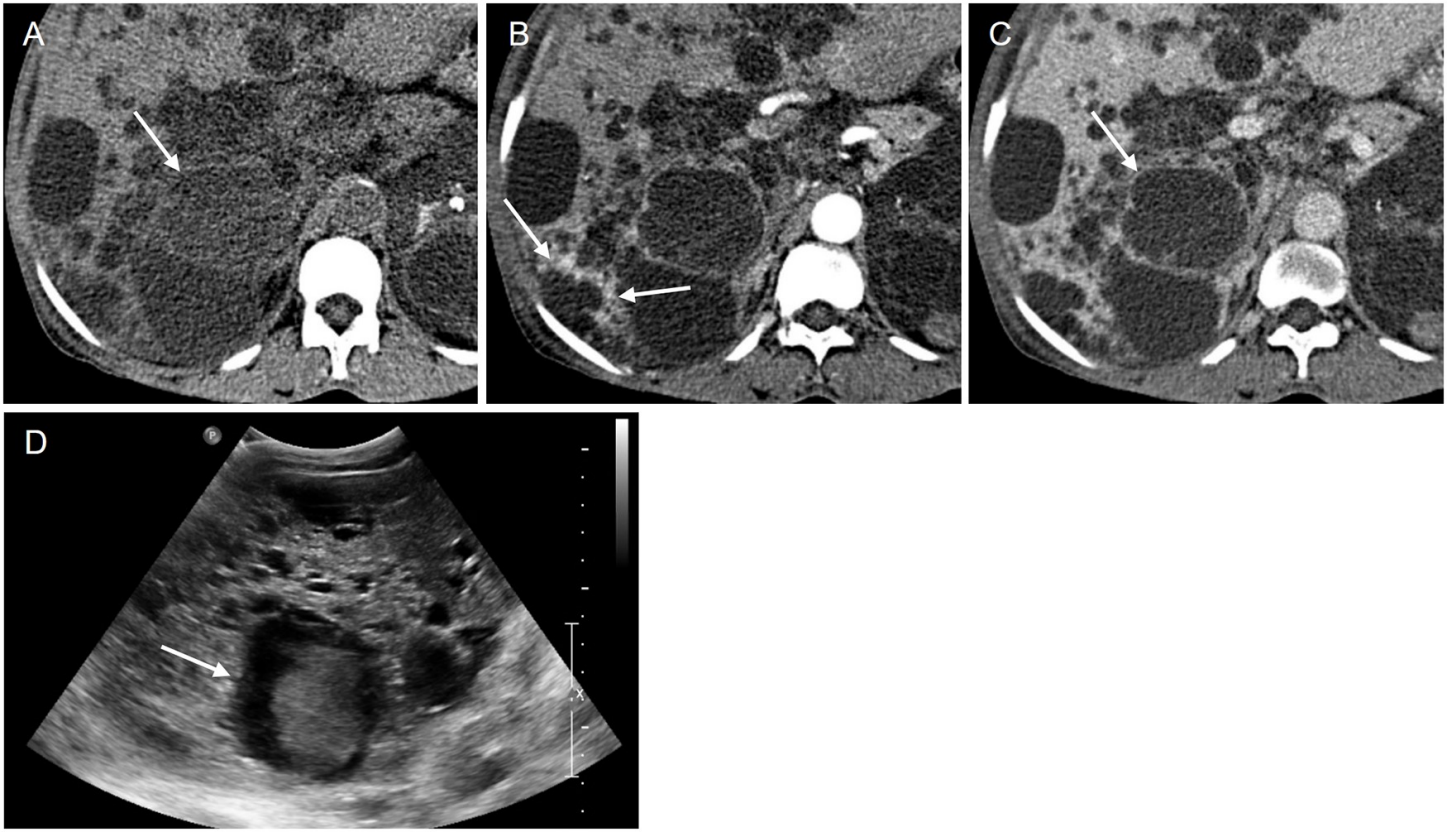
A 59-year-old female patient with an infected cyst (H12 in Fig 2). (A) The pre-contrast CT image shows a 5.5 cm sized cyst with a lobulated contour in segment 6 of the liver (arrow). The cyst appears to have a relatively high attenuation compared to the surrounding cysts. (B) The arterial phase image shows arterial hyperenhancement (arrows) in segment 6 of the liver; however, perilesional hyperemia could not be definitely diagnosed due to the intervening cysts between the lesion and hyperemia site. (C) The cyst shows discernible wall thickening in the portal venous phase. Of note, intracystic attenuation seems equal in contrast to the pre-contrast scan. (D) Ultrasonography shows a heterogeneous hyperechoic cyst with internal septation (arrow).
